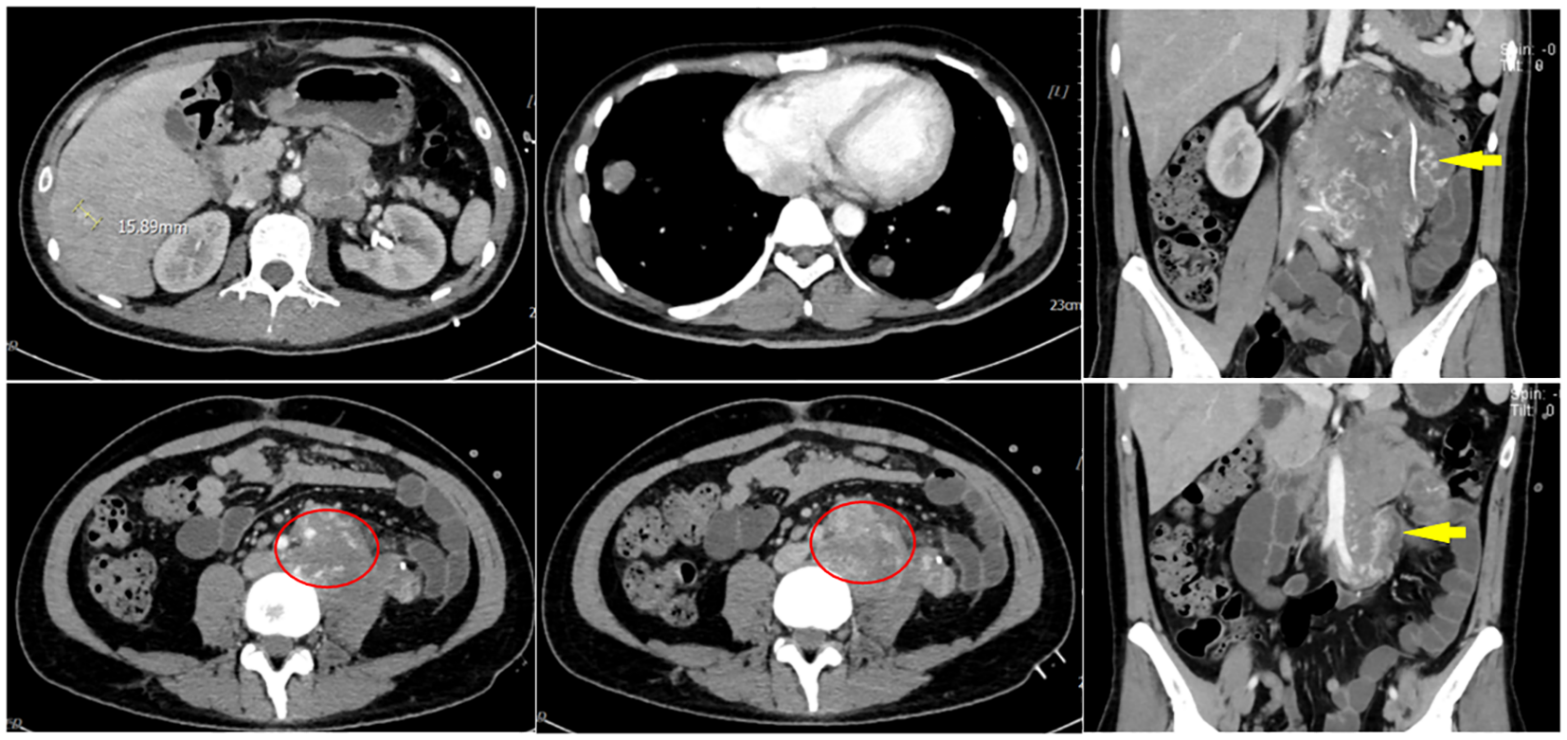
On March 1, 2023, an abdominal CT scan revealed irregular mixed-density masses in the retroperitoneum and left abdominal cavity, measuring 66 mm × 82 mm and 46 mm × 44 mm, respectively. These masses surrounded the abdominal aorta, extended below the bifurcation, and affected the left psoas major muscle and ureter definition. Heterogeneous enhancement in the mass with migrating vascular shadows was observed, along with multiple lymph nodes in the left abdominal cavity and a nodular omentum. Additionally, multiple intensifying nodules were noted in the right hepatic lobe during the arterial phase, with isodensity in the portal and delayed phases. No fluid was present in the abdominal cavity, but enlargement of retroperitoneal lymph nodes and multiple nodules in both lungs were suspected to be metastatic.
